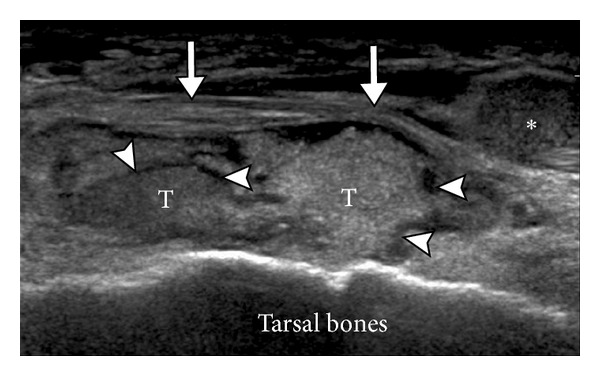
Tophus in gout. Ultrasound appearance of a tophus (T) overlying the dorsal aspect of the tarsal bones and underlying the extensor digitorum tendons (long arrows). Note the anechoic peripheral halo (arrowheads) and hyperechoic heterogeneous center. *Echogenic fluid.
